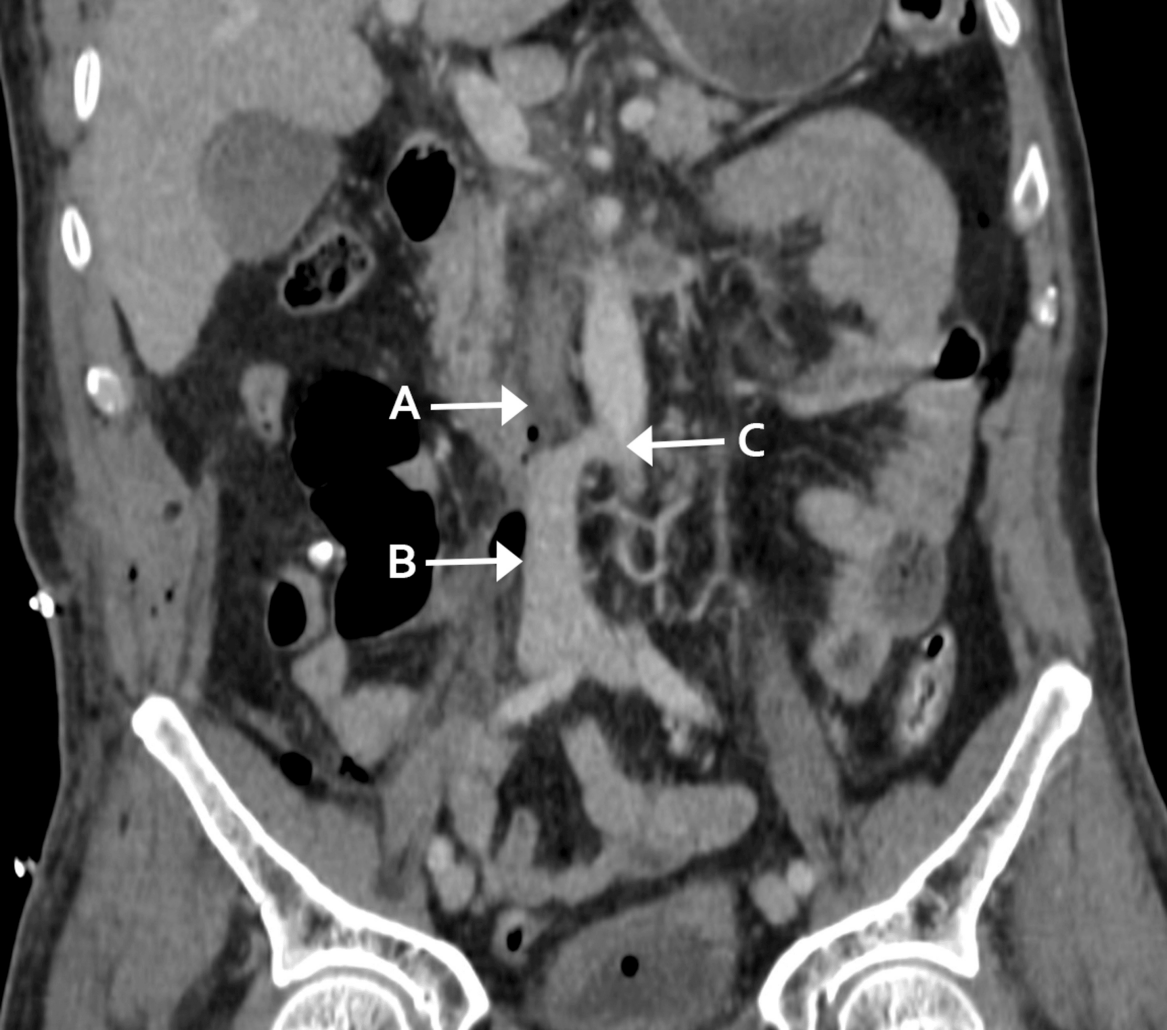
Contrast-enhanced abdominal CT, coronal section. Arrows: A: IVC occlusion; B: IVC; C: azygos vein IVC, inferior vena cava
